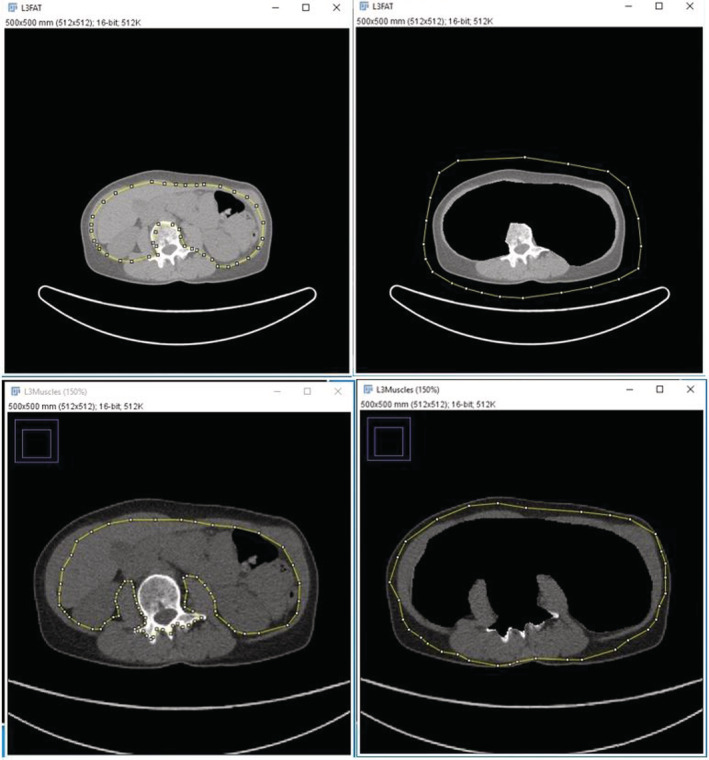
Examples of skeletal muscle contours. Pictures are example contours of segment manually muscles, subcutaneous adipose tissue and visceral adipose tissue at level L3 on computed tomography.
